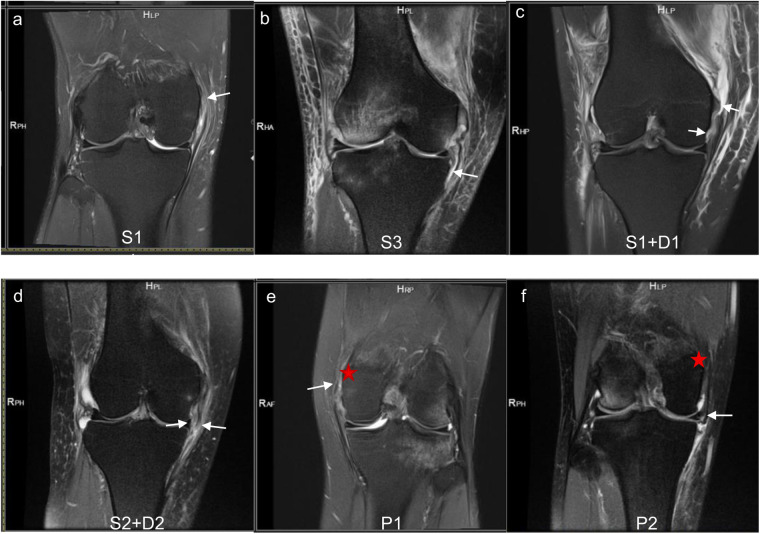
Coronal fat-saturated proton density-weighted (PDFS) magnetic resonance images demonstrate the tear areas of the medial collateral ligament (MCL). Image (a) shows a tear at the femoral side of the superficial medial collateral ligament (sMCL) (solid arrow). Image (b) reveals a tear at the distal tibial side of the sMCL (solid arrow). Image (c) demonstrates a combined tear at the femoral sides of both the sMCL and deep medial collateral ligament (dMCL) (solid arrow). Image (d) shows a concurrent tear at the proximal tibial sides of both the sMCL and dMCL (solid arrow). Image (e) shows a tear at the femoral side of the POL (solid arrow). (f) displays a tear at the tibial side of the POL (solid arrow). The adductor tubercle is indicated by an asterisk.
